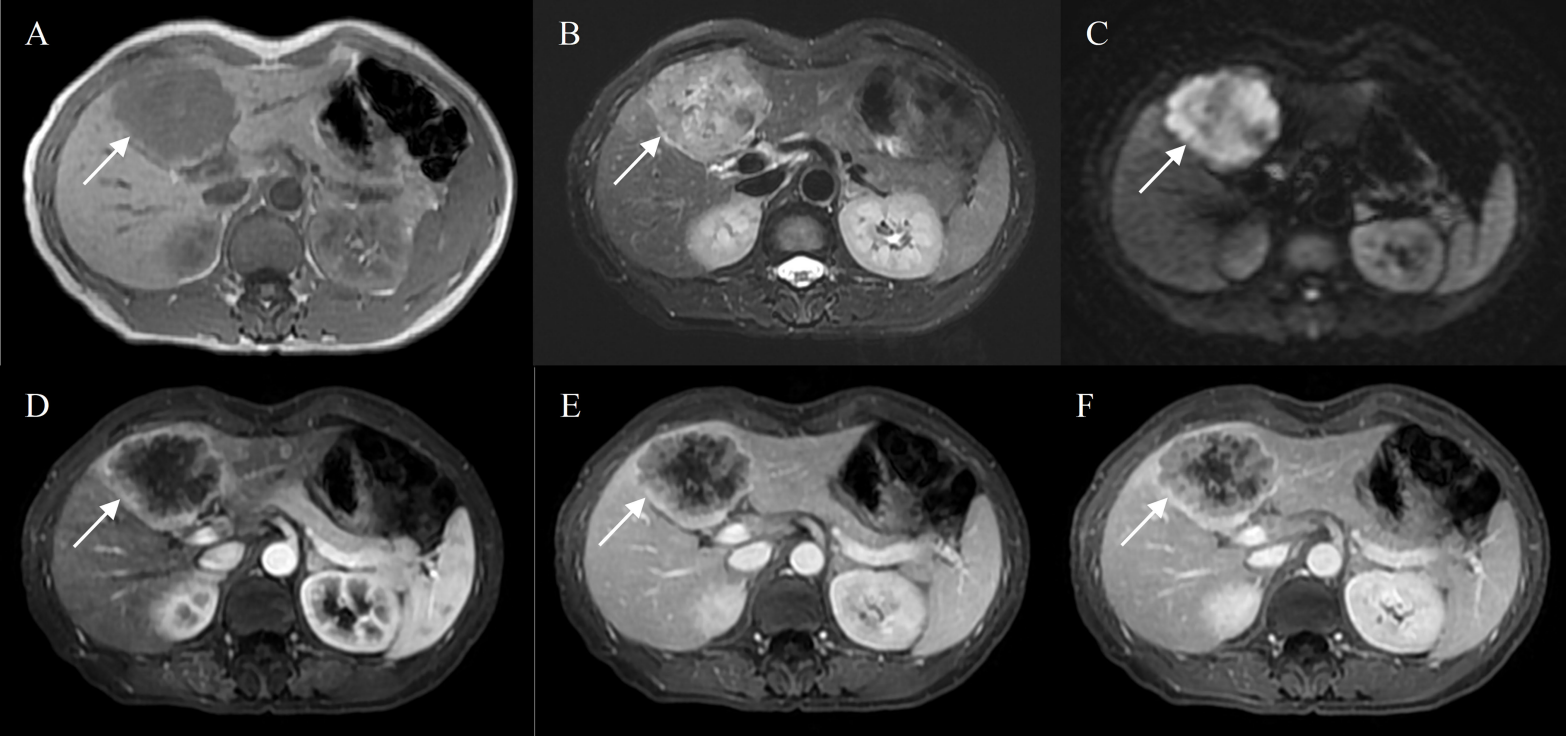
Intrahepatic mass-forming cholangiocarcinoma in a 50-year-old female with hepatitis B virus. Gd-DTPA-enhanced MRI detected a lobulated tumor (7.0 cm) with heterogeneous SI on T1WI and T2WI (A, B), restricted diffusion (C). Peripheral rim-like enhancement in the arterial phase (D), followed by progressive filling of the contrast material in the portal and delayed phase (E, F).
